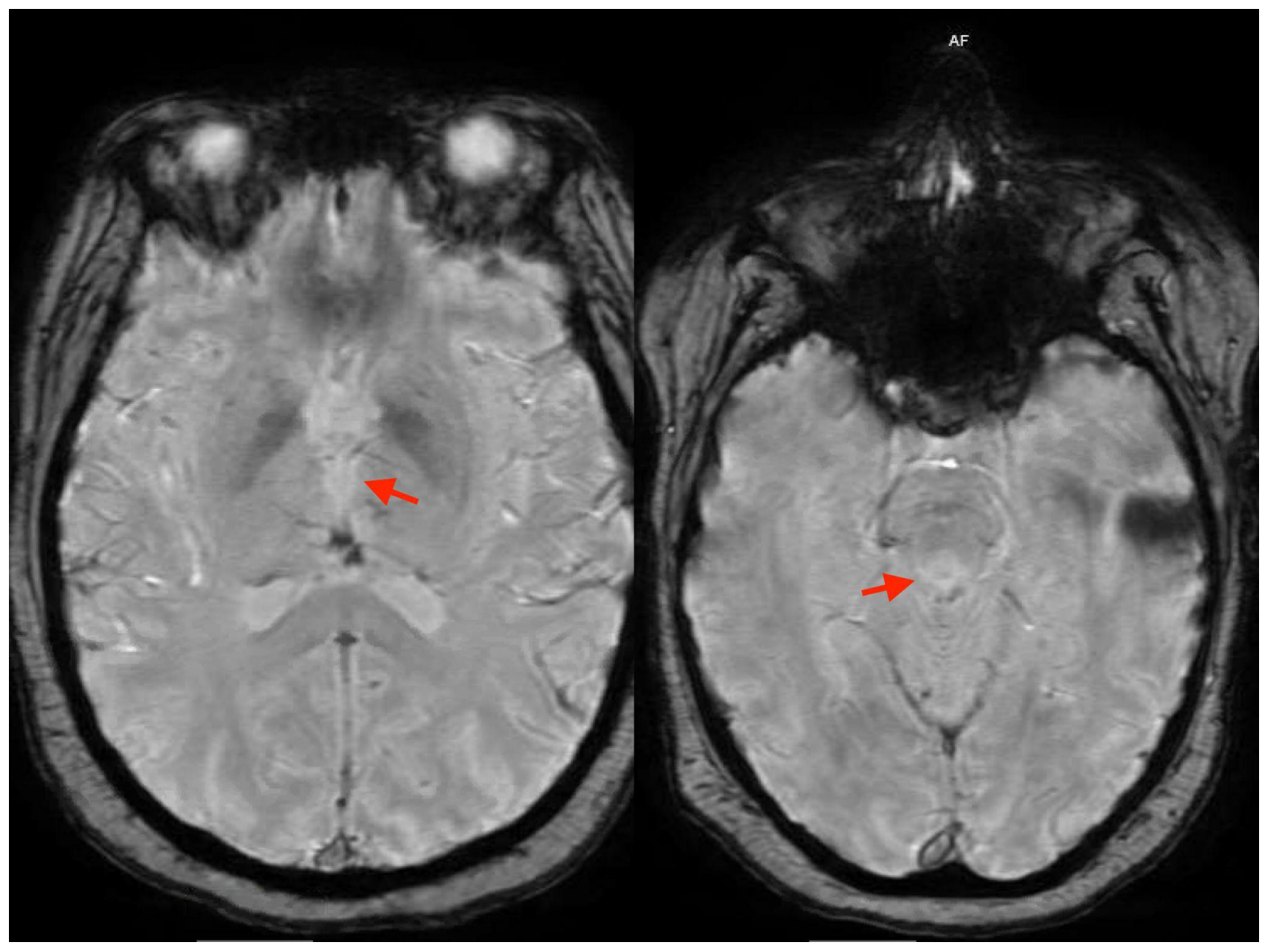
Signal changes in the bilateral medial thalami (left) and mammillary bodies (right) on MRI.
